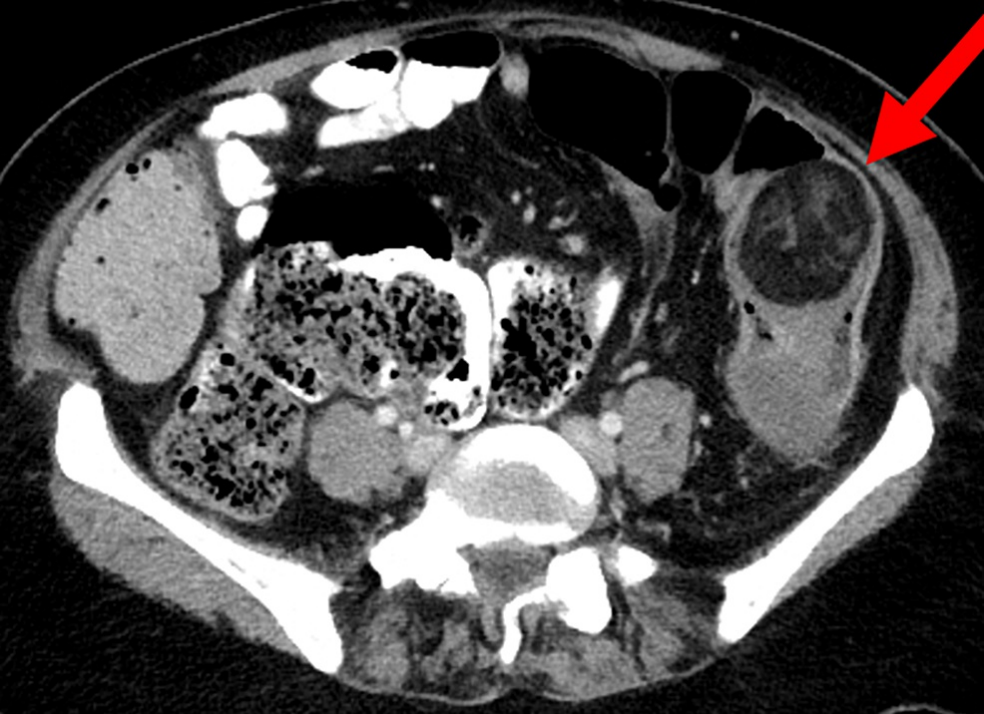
Axial CT scan showing lipoma
